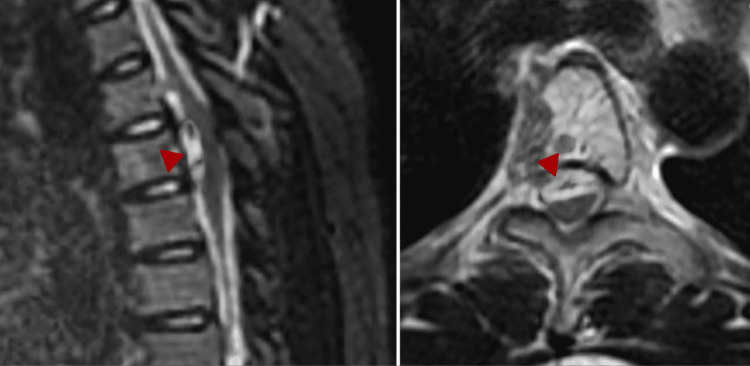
Sagittal (left) and axial (right) T2 magnetic resonance image of thoracic spine demonstrating compressive T4 lesion
